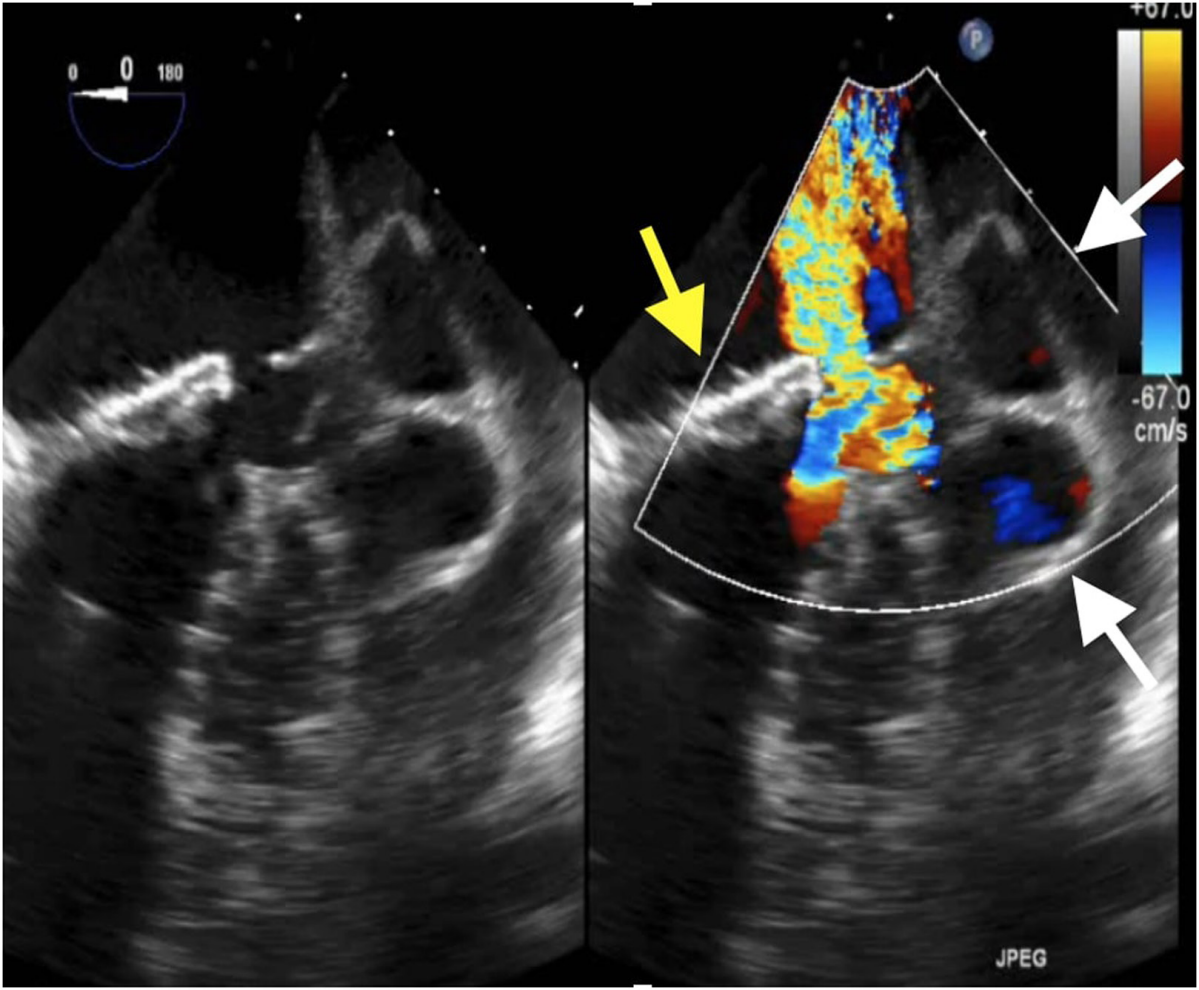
Transesophageal echocardiography showing the partially detached mitral prosthetic valve with mitral valve regurgitation (yellow arrow), and left ventricular pseudoaneurysm with color Doppler flow (white arrow)
